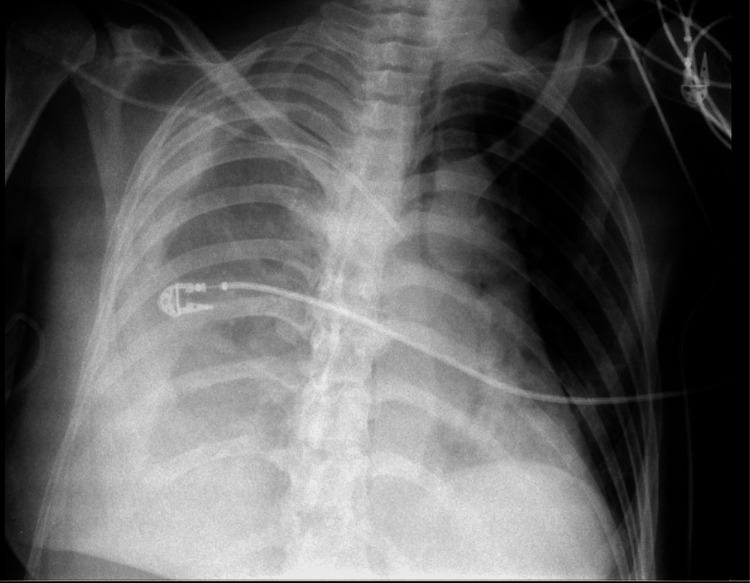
Anteroposterior chest X-ray showing reduced transparency of the right hemithorax, suggestive of pleural effusion
